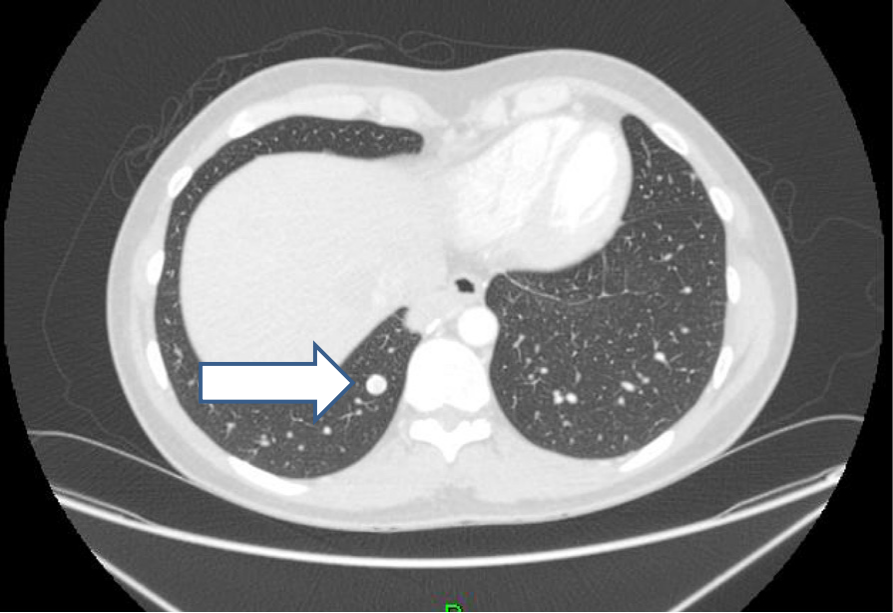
CT image of a lung nodule.
